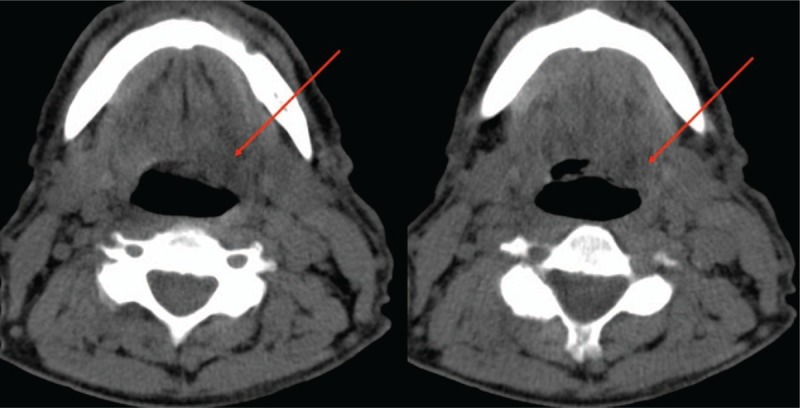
CT (without contrast) scan on 17 December 2014 of the vocal cords shows tissue of higher density (arrows). CT = computed tomography.
